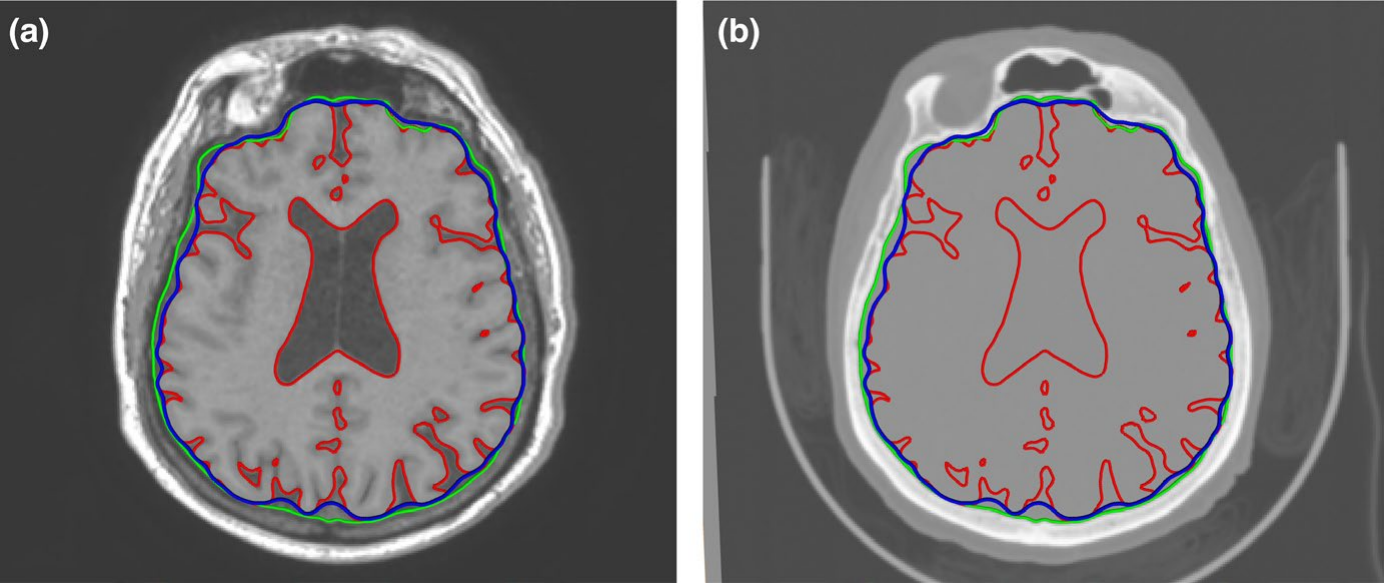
Registration of the images from the MRIs (a) and CT scans (b) with the segmentation of the brain (red, generated from the MRIs and transferred to the CT scans), brain hull (blue, generated from the MRIs and transferred to the CT scans) and endocast (green, generated from the CT scans and transferred to the MRIs) being superimposed. The patient lays supine
